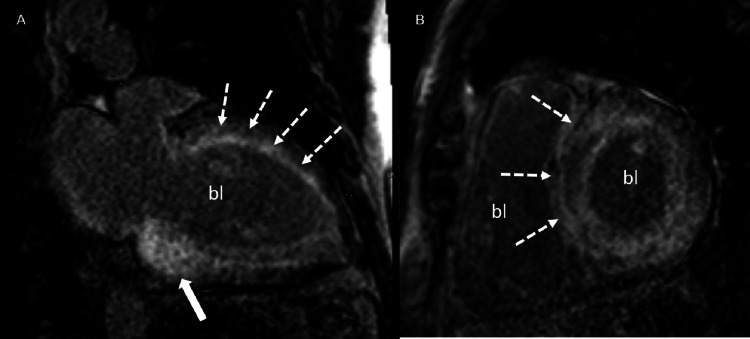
Cardiac magnetic resonance imaging Vertical long-axis (A) and short-axis (B) phase-sensitive post-contrast inversion recovery images demonstrate nonterritorial diffuse subendocardial enhancement (dotted arrows) characteristics of CA. Notice the loss of blood pool signal (bl) due to high circulating levels of amyloid. Note the somewhat confluent and prominent involvement at the base (solid arrow) and relative sparing of the left ventricular apex, another feature of CA.
